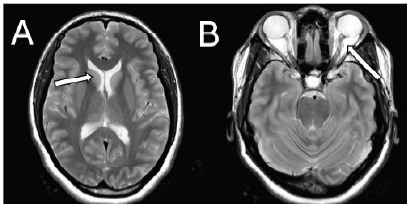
Magnetic resonance imaging demonstrating slit ventricles (white arrow in A) and tortuous optic nerve (white arrow in B).
